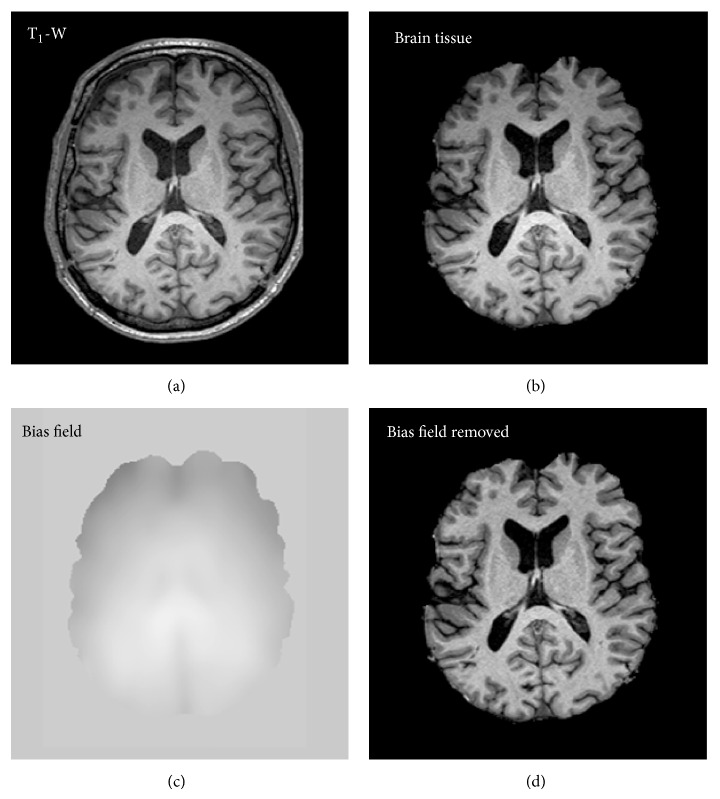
Preprocessing steps: (a) the original T_1_-W MR image of the adult brain; (b) the brain tissue image after removing nonbrain structures; (c) the bias field; (d) the brain tissue image after bias field correction.
